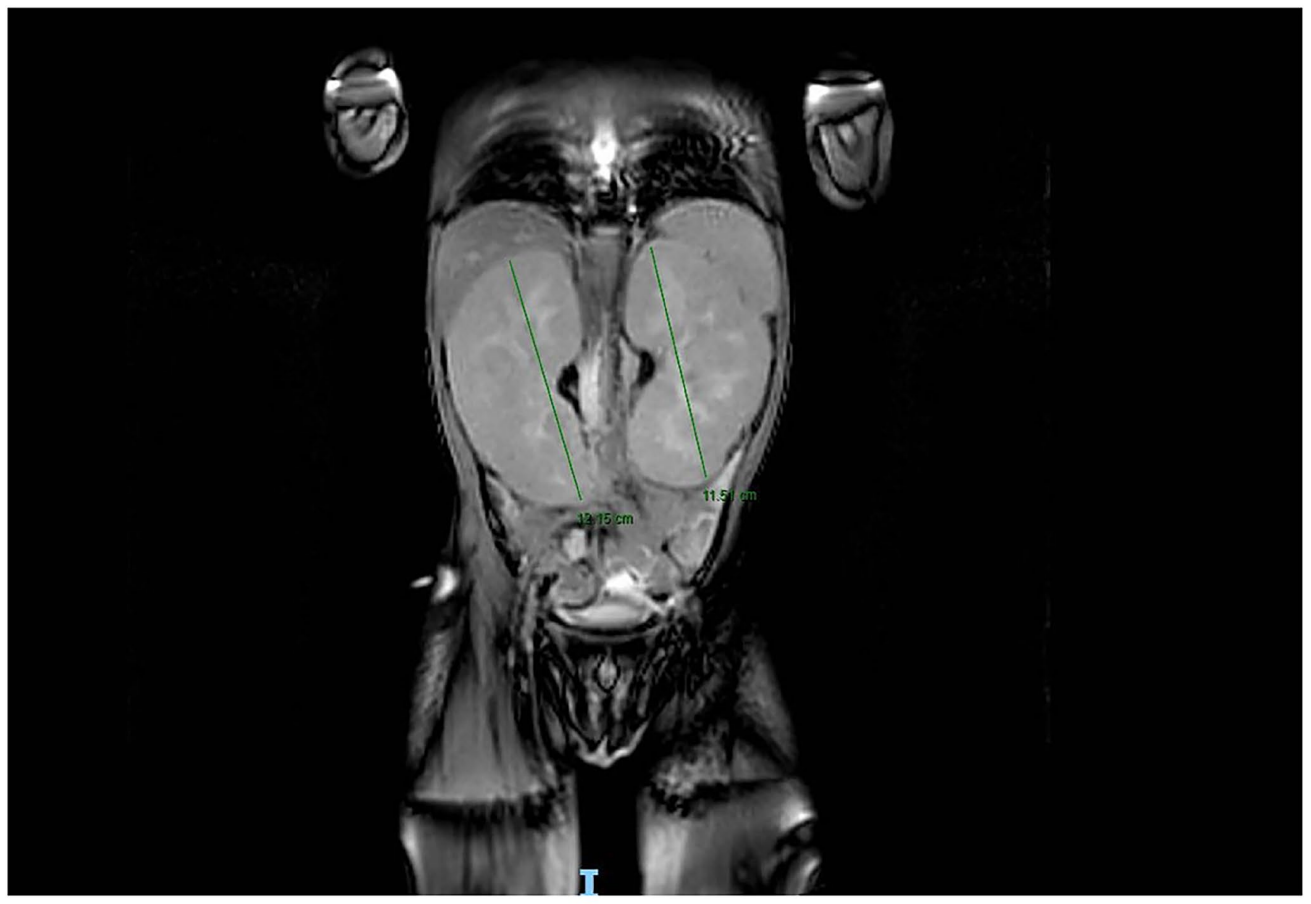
Abdominal MRI. Bilateral nephromegaly noted, with the right kidney measuring 12.15 cm and the left 11.51 cm.
